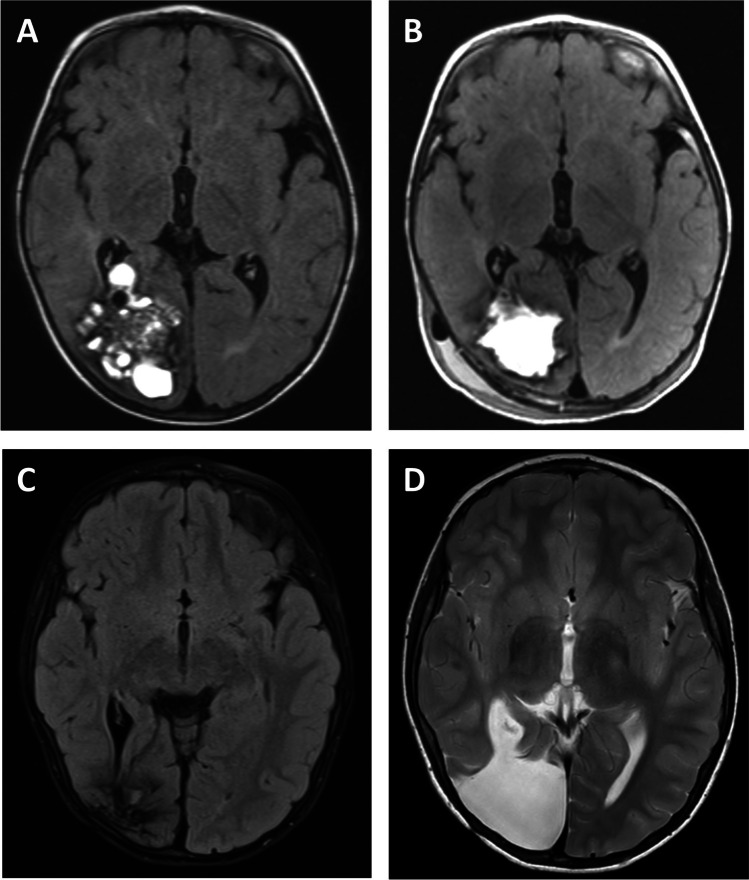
In a male 1.5-year-old patient who was exhibiting seizures, a large right occipital CM was resected adjacent to the area of the visual cortex (A). As shown in B, resection was restricted to the CM itself without radical removal of the hemosiderin rim. Follow-up MRI showed persisting hemosiderin deposits in the cortex around the resection cavity (C). After extension of the initial resection, which included all hemosiderin remnants, the patient achieved seizure-freedom (D)
